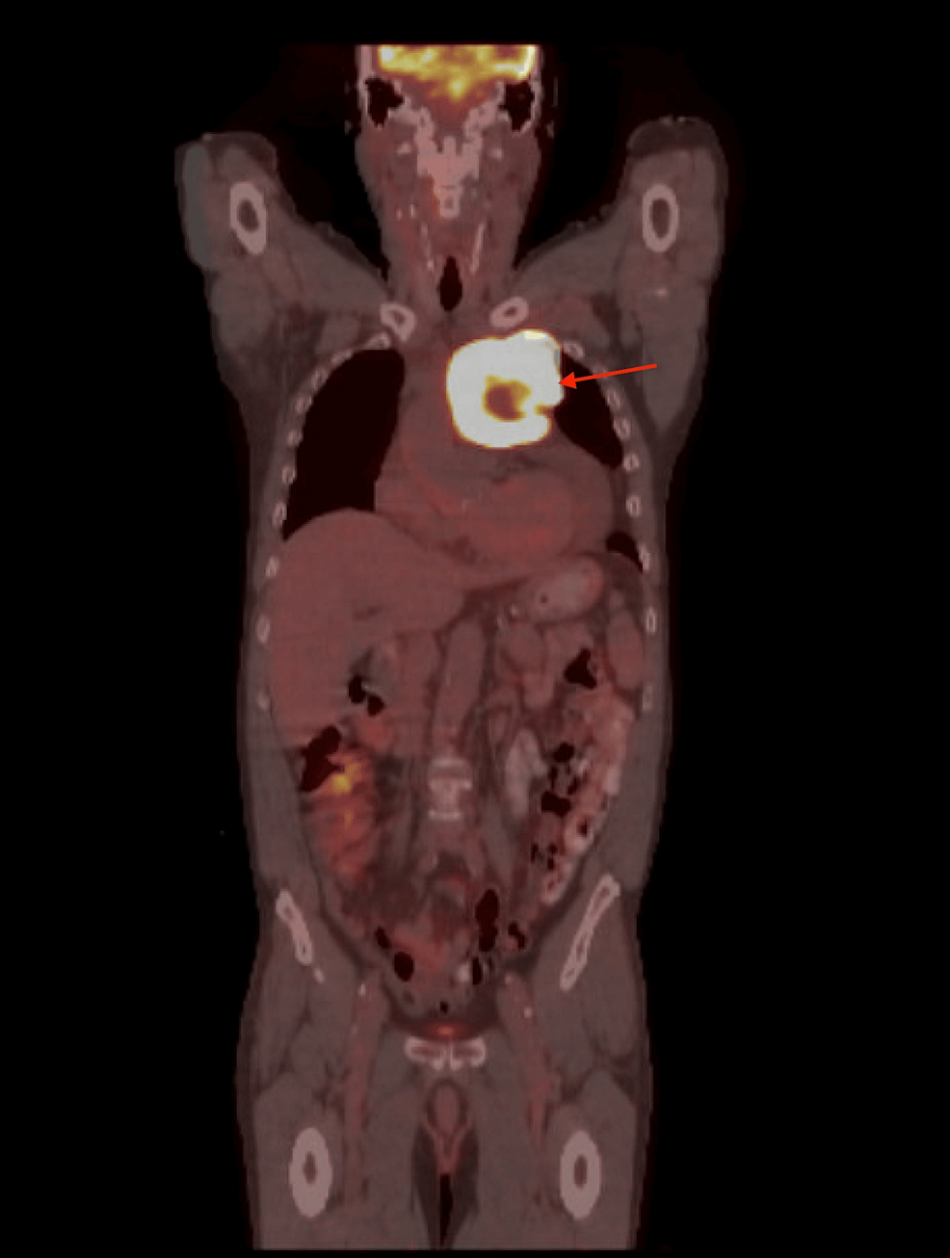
PET scan of the patient The image shows a large FDG-avid left anterior mediastinal mass with increased FDG activity peripherally with max SUV of 24.0 (red arrow) and decreased activity centrally suggestive of malignancy with central necrosis FDG: fluorodeoxyglucose; PET: positron emission tomography; SUV: standardized uptake values
